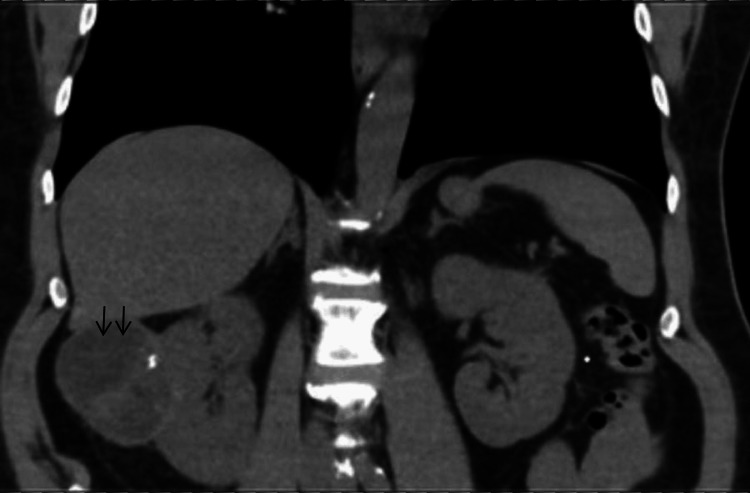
Abdominopelvic CT demonstrates a large multiloculated cystic lesion in the right kidney, with internal septations and calcifications, consistent with a complex renal cyst (arrows)
